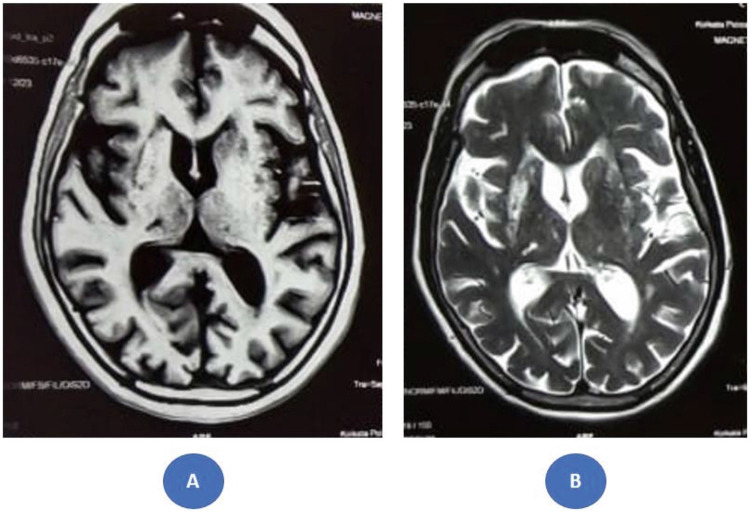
(A) Magnetic resonance imaging showing subtle T1 hyperintensity in the right striatum after 1 year of follow-up, with no appreciable signal alteration on the left side. (B) Corresponding T2 images displaying bilateral striatal hyperintensity (right > left) along with more pronounced putaminal atrophy on the right side.
